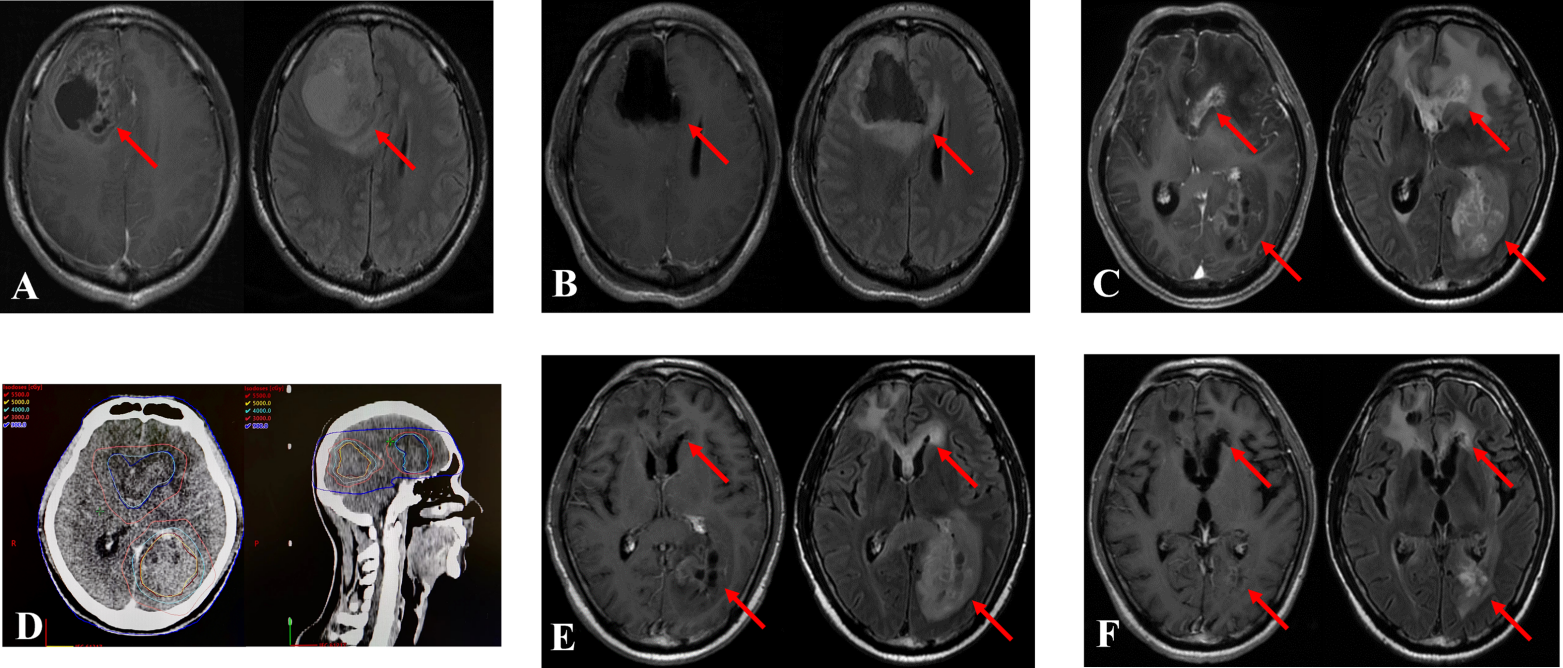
Treatment case of re-irradiation therapy. The two recurrent tumors were diagnosed in a 39-year-old man 13.1 months after the initial operation. The patient underwent re-irradiation of 40 Gy in 20 fractions at *in situ* focus and 50 Gy in 25 fractions at distant locus. (A): Magnetic resonance imaging (MRI) at initial diagnosis. Left: T1 enhanced MRI, Right: T2 fluid enhanced MRI. (B): MRI after the operation. (C): MRI at recurrence. (D): Re-irradiation treatment plan. (E): MRI after re-irradiation. (F): MRI 6 months after re-irradiation. The arrow indicates the tumor area.
